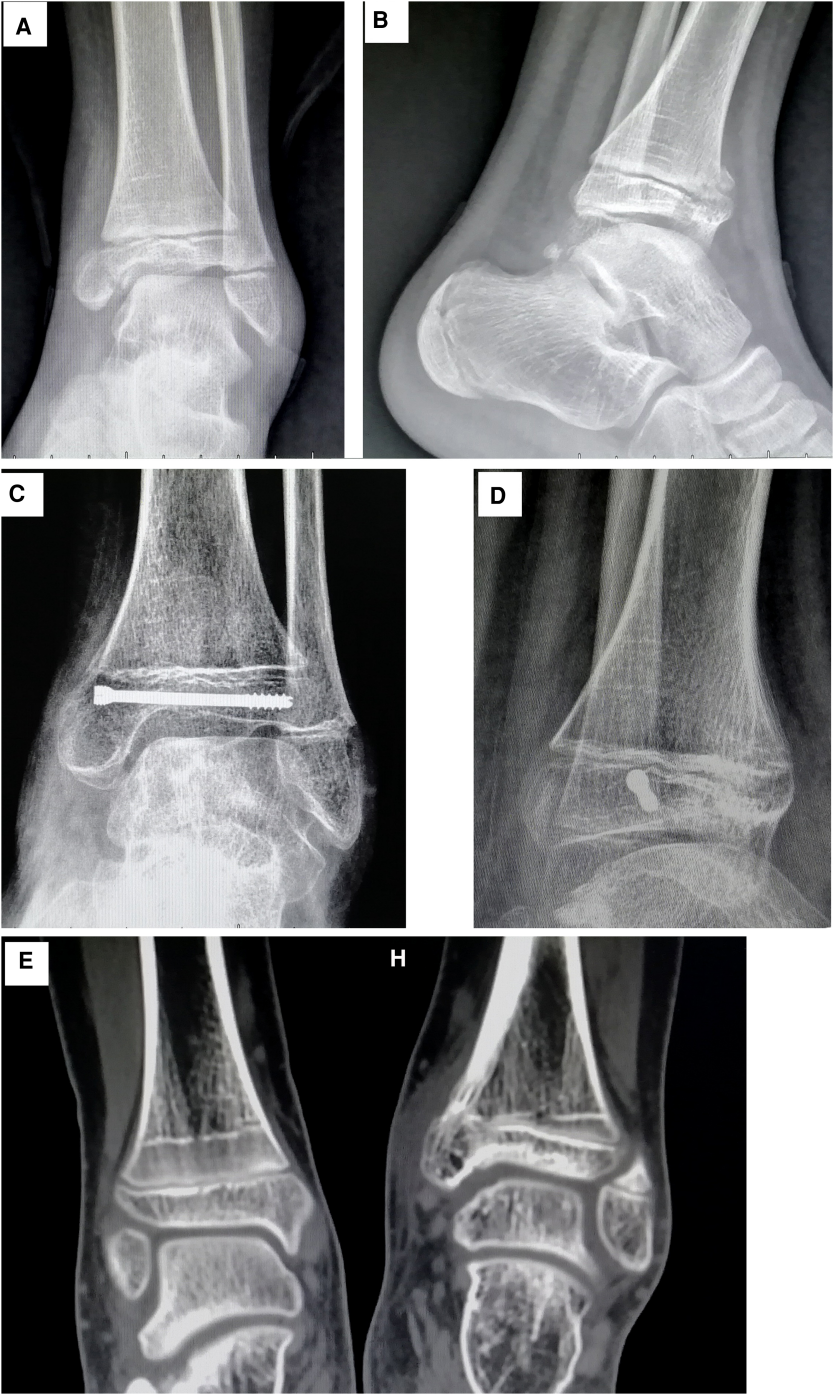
(A) Radiographs and CT images of a 12-year-old boy. The anteroposterior view shows a tibial epiphyseal fracture on the left side. (B) Lateral view showing that the fracture line crosses the epiphyseal plate (Salter–Harris type IV fracture). (C) Radiograph taken after 90 days of follow-up showing the growth arrest line. Formation of the medial bone bridge also can be seen. (D) Lateral view showing the bone bridge in the front of the epiphysis. (E) CT scan of bilateral ankle joints showing that the growth arrest line of the healthy side is parallel to the epiphysis, whereas the growth arrest line of the affected side forms an angle with the epiphysis. The apex of the angle is at the medial bone bridge.
